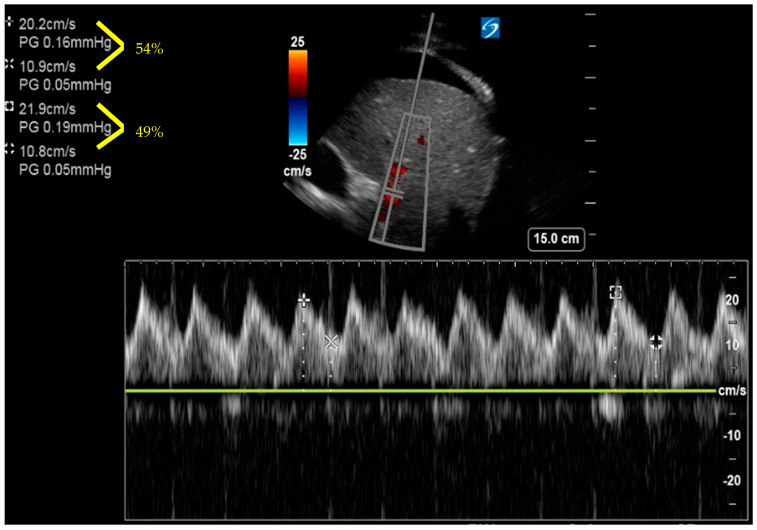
Doppler of portal vein showing >50% pulsatility index.
